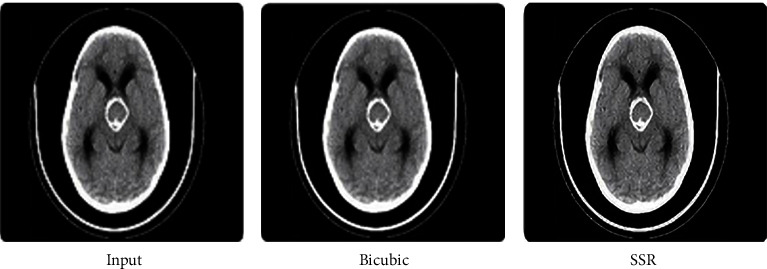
Comparison of input, bicubic, and our SSR of sagittal brain MRI.
